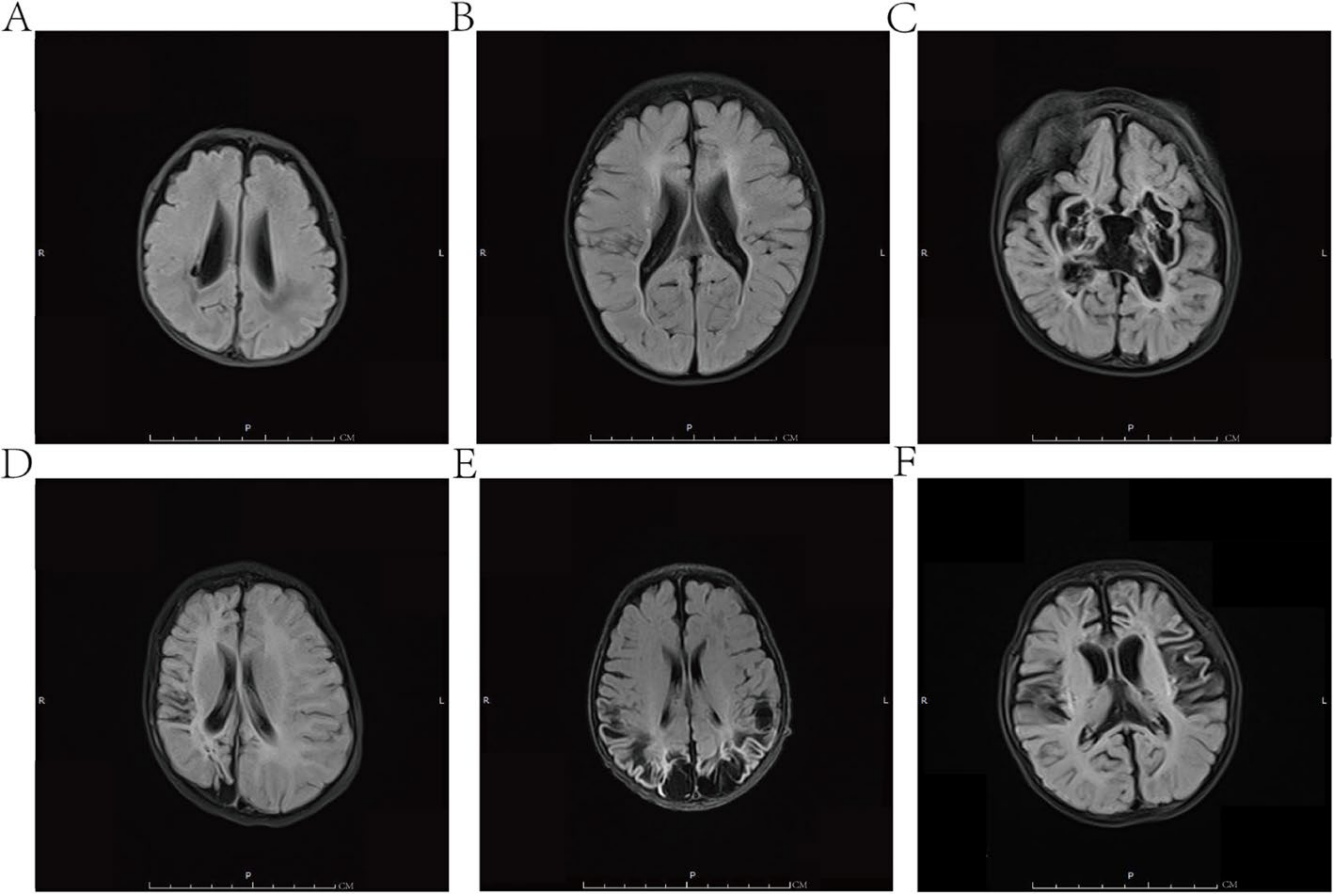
Different types of leukomalacia on head MRI (T2 FLAIR). A. Grade I PVL: local changes in the small capsule cavity around the body of the right lateral ventricle. B. Grade II PVL: extensive cystic changes around the ventricle, with an irregular ventricular wall and ventricular dilatation. C. Grade III PVL: cystic changes in the periventricular and subcortical white matter. D. SCL: cysts in the right parietal lobe. E. SCL: cysts in the bilateral parietal and occipital lobes. F. SCL: cysts in the bilateral frontal and parietal lobes
